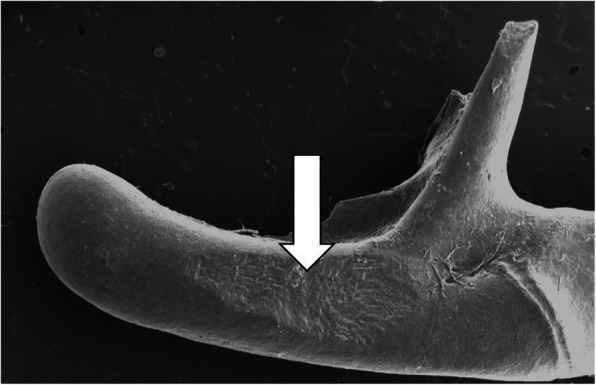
SEM of malleus showing the extent of bone removal after a 30s application of the with ultrasonic bone removal device
